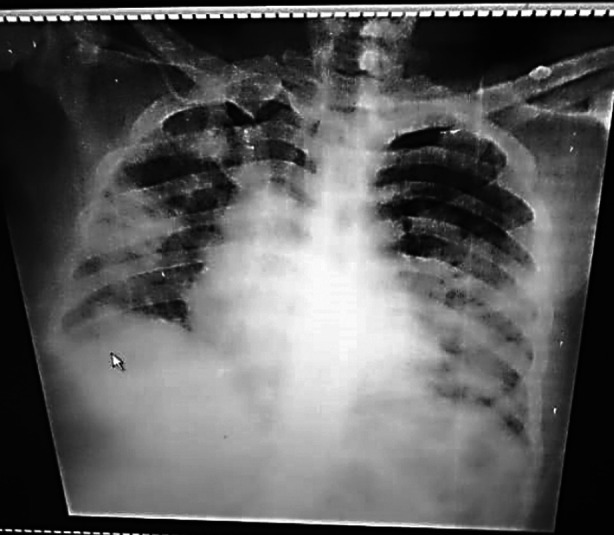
49 years old male patient chest X-ray on presentation showed right three zone and left two zone heterogeneous predominant peripheral opacities.
